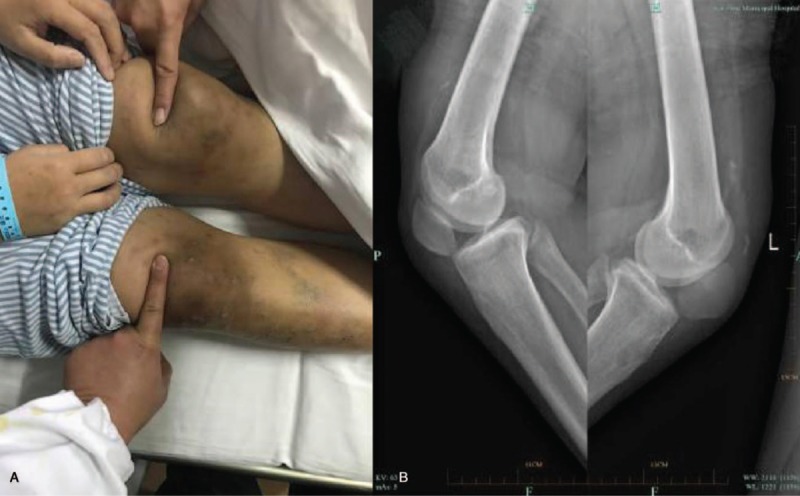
(A) Photograph of both knees revealing the bilateral soft tissue gap in the suprapatellar region. (B) Bilateral lateral radiography of her knees revealed inferior displacement of both patellas with irregular calcified deposits in the suprapatellar region (quadriceps tendon).
